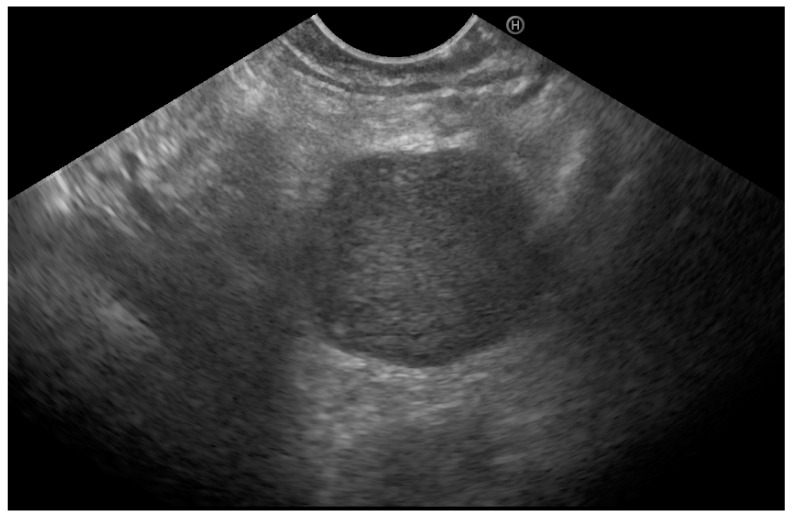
Endoscopic ultrasound using a linear probe positioned in the duodenum, showing a hypoechoic round mass in the head of the pancreas.
